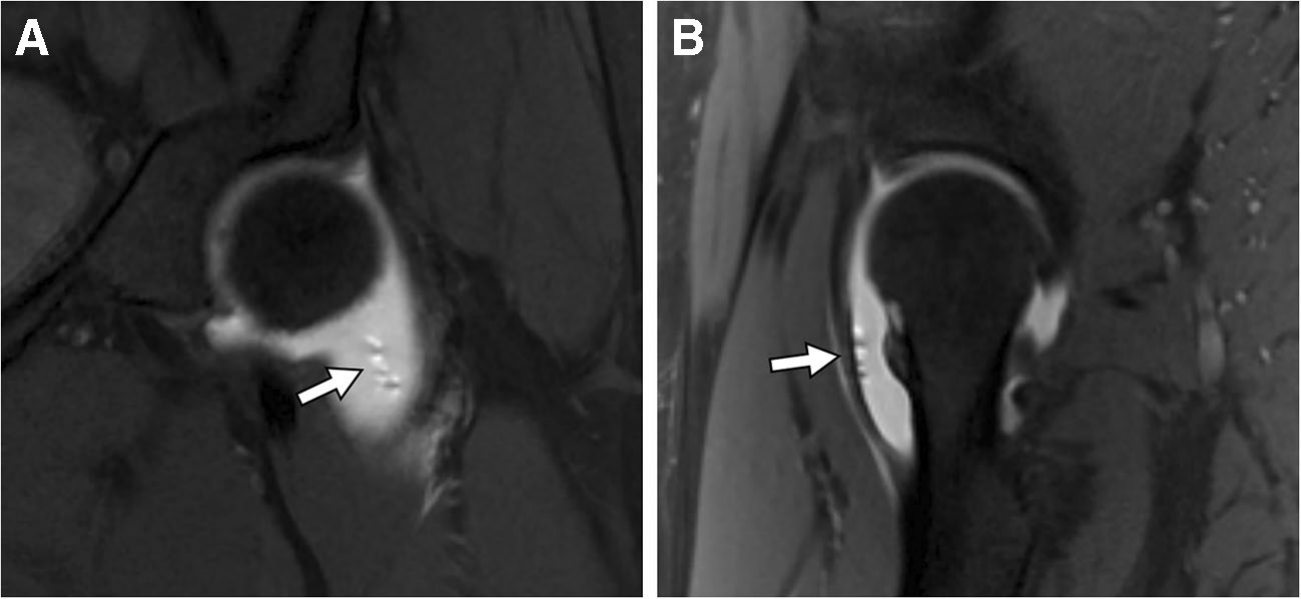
Intra-articular bubbles. Forty-nine-year-old woman with hip pain. Coronal (A) and sagittal (B) T1-weighted fat-suppressed 3 T MR arthrogram images show bubbles in the nondependent portion of the joint causing a dipole field pattern artifact (arrows)
